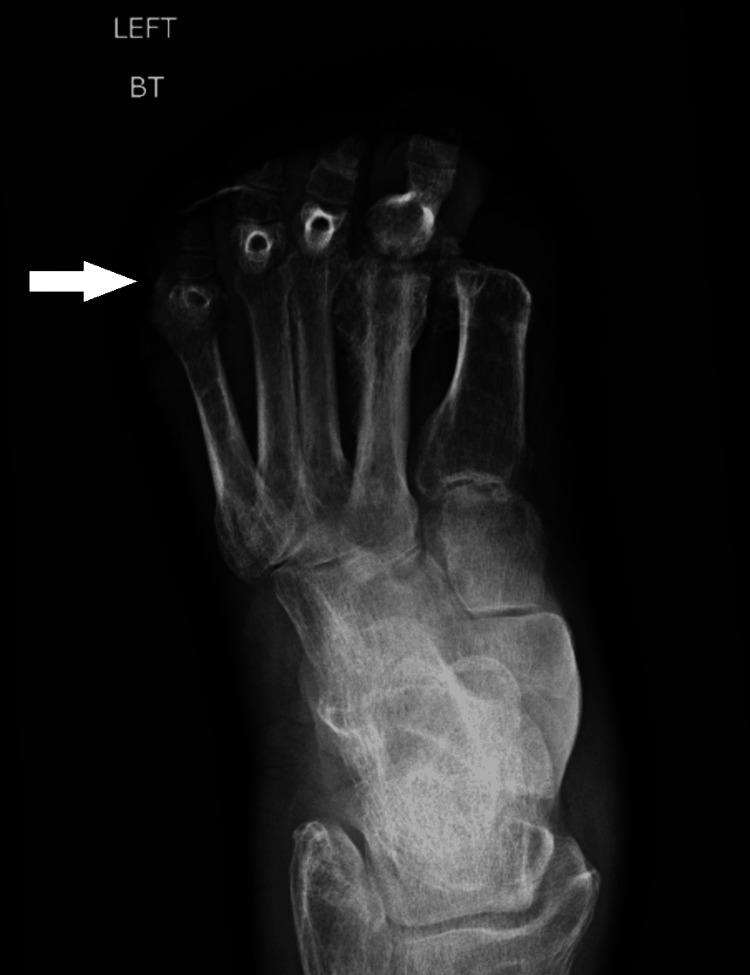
X-ray of the left foot Anterior-posterior (AP) view of the left foot shows prior amputation of the first distal phalanx in addition to cortical irregularity at the base of the fifth proximal phalanx (marked by the arrow).
